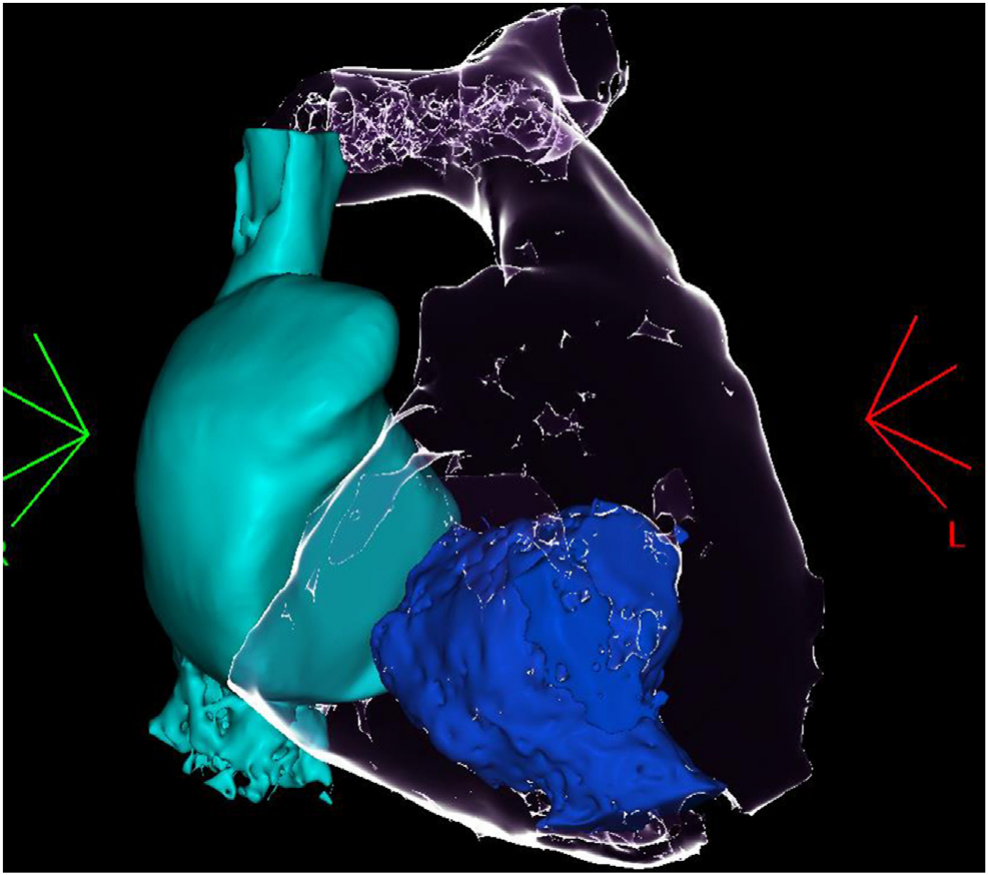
Computed tomography—3-dimensional reconstruction of the right heart chamber. Light blue, right atrium; dark blue, right ventricul mass; transparent, right ventricle.
